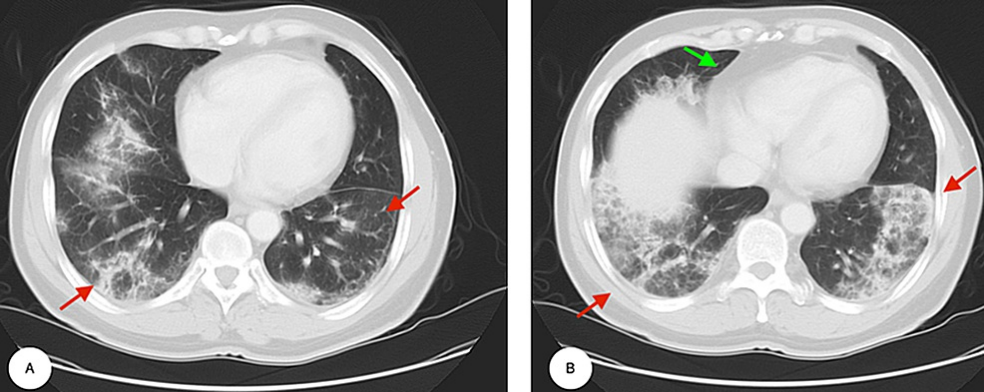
Multi-slice computed tomography (CT) of the chest. (A) Horizontal tomography slice of the mid thorax and (B) lower thorax demonstrating interlobular reticular septal thickening and ground-glass opacity in bilateral mid and lower lung lobes (red arrows) with mediastinal lymphadenopathy (green arrow).
